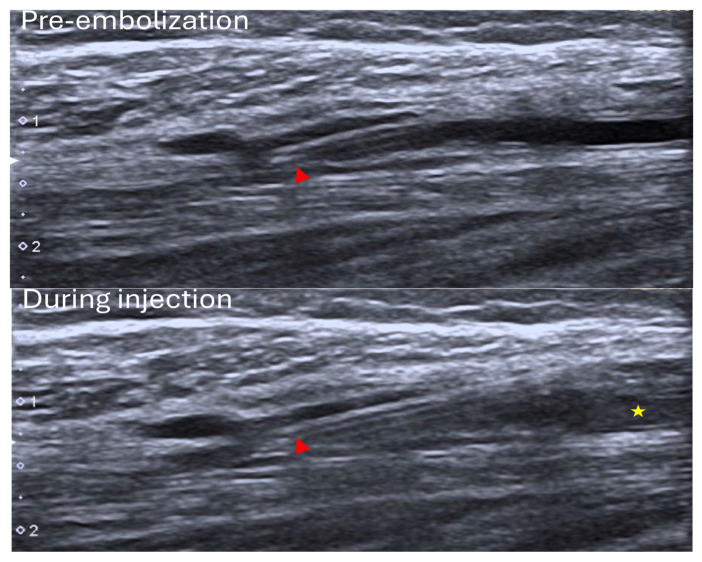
Ultrasound images acquired before embolization (top) and during imipenem–cilastatin injection (bottom). The needle tip is indicated by the red arrowhead. During injection, the appearance of intraluminal hypoechogenicity (yellow star) reflects the presence of imipenem/cilastatin, confirming intravascular delivery.
